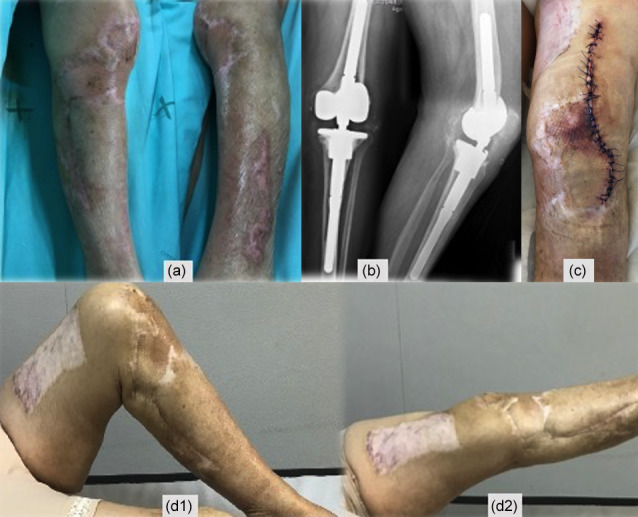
(a) Skin before the second-stage surgery, (b) post-operative X-ray, (c) skin fully
